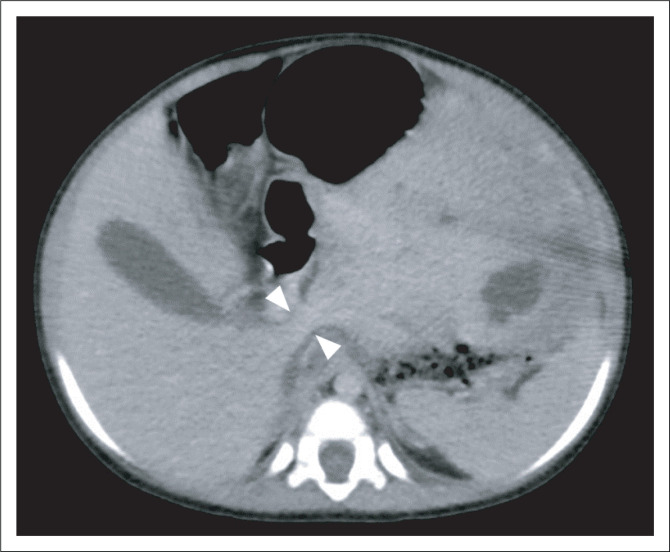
Follow-up imaging – Axial contrast-enhanced computed tomography of the abdomen (portal venous phase) shows the mass within the left upper abdomen. A thin pedicle is seen connecting the mass to the caudate lobe of the liver (arrowheads). This confirms a pedunculated mass of hepatic origin.
